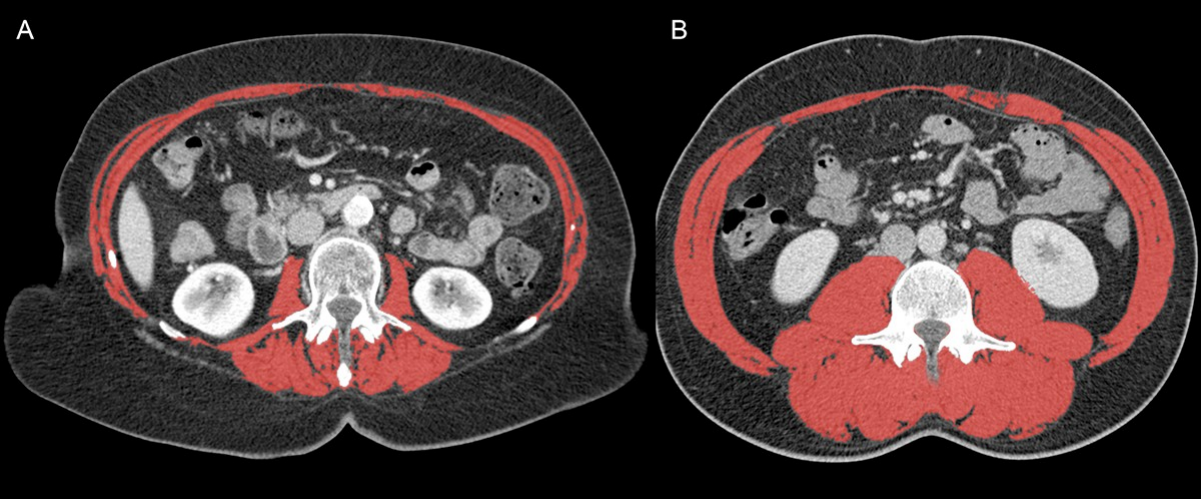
Determination of body composition in patients with resectable pancreatic ductal adenocarcinoma. Axial contrast-enhanced portal-venous phase CT image at the L3 level of a sarcopenic obese patient (A, 67-year old female, BMI 33 kg/m^2^, total muscle tissue cross-sectional area 109.2 cm^2^, skeletal muscle index 39.3 cm^2^/m^2^) compared to a non-sarcopenic obese patient (B, 71-year old female, BMI 31 kg/m^2^, total muscle tissue cross-sectional area 130.4 cm^2^, skeletal muscle index 47.7 cm^2^/m^2^). Marked red: psoas, paraspinal, transverse abdominal, external oblique, internal oblique and rectus abdominis muscles.
